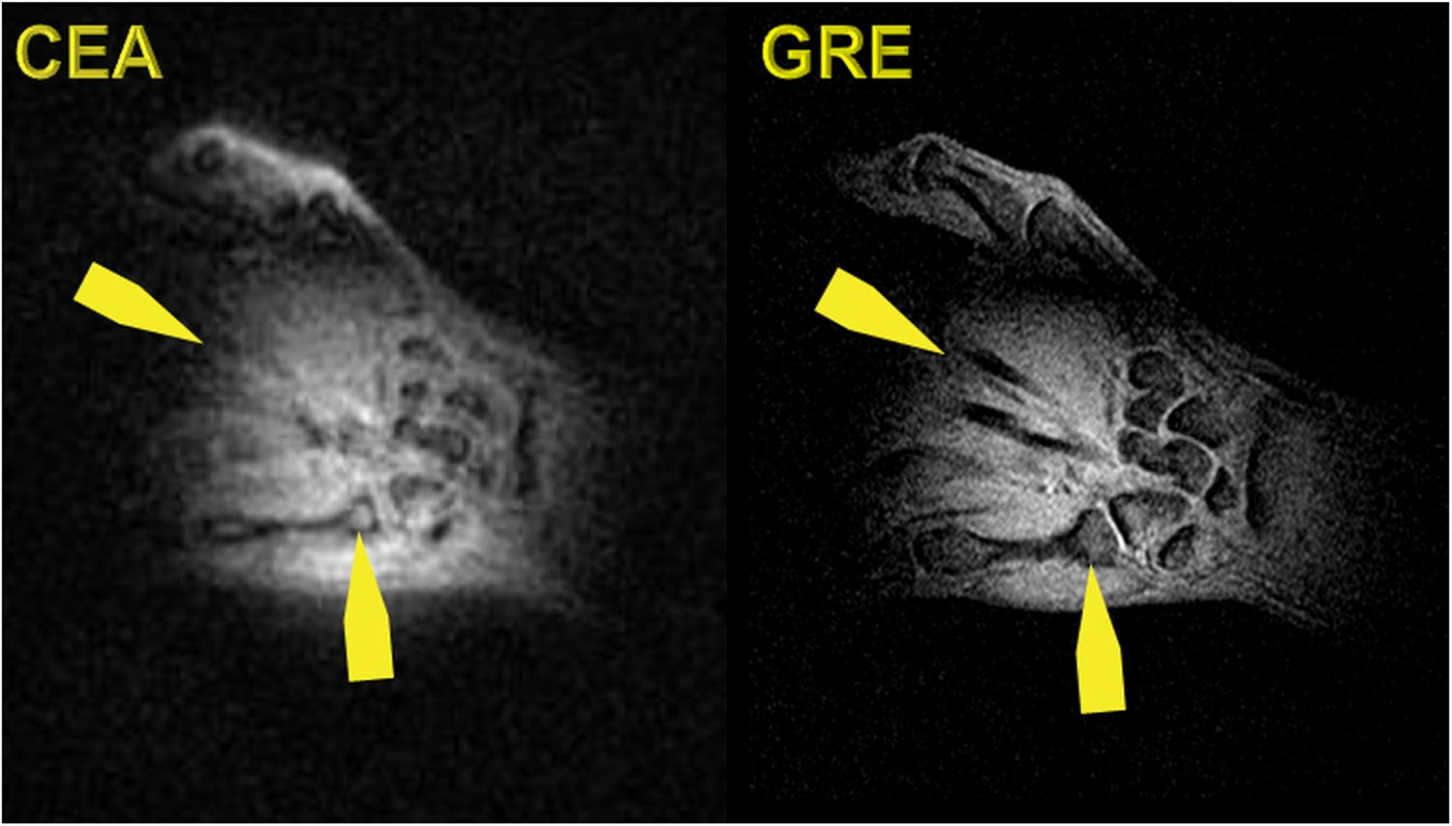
Coronal slice from the 3D CEA MR image of the healthy volunteer wrist (left), and GRE image as the anatomical reference (right).
